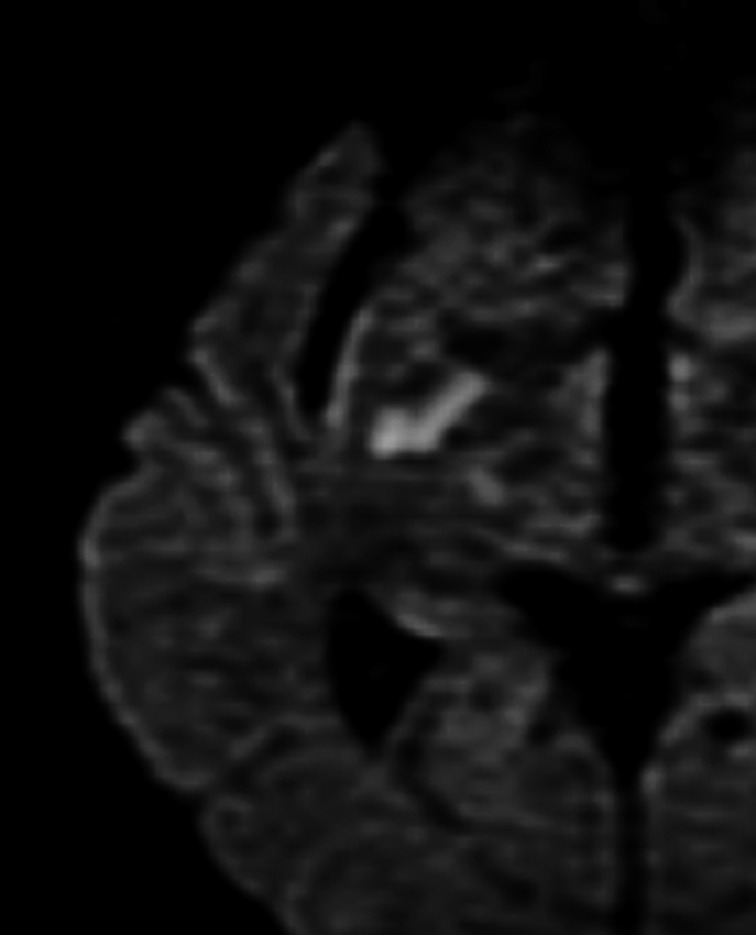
Small ischemic strokes seen on DWI MRI affecting inferior posterior basal ganglia as well as a smaller right lateral inferior posterior thalamus at the vicinity of LGN
